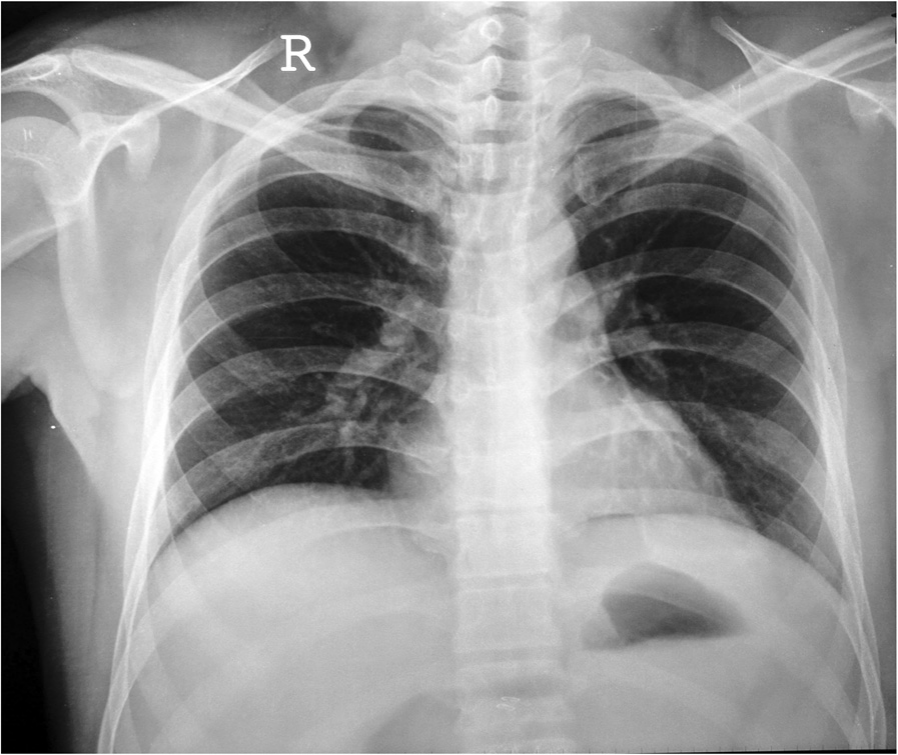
X-ray chest showing full expansion of the left lung on discharge
